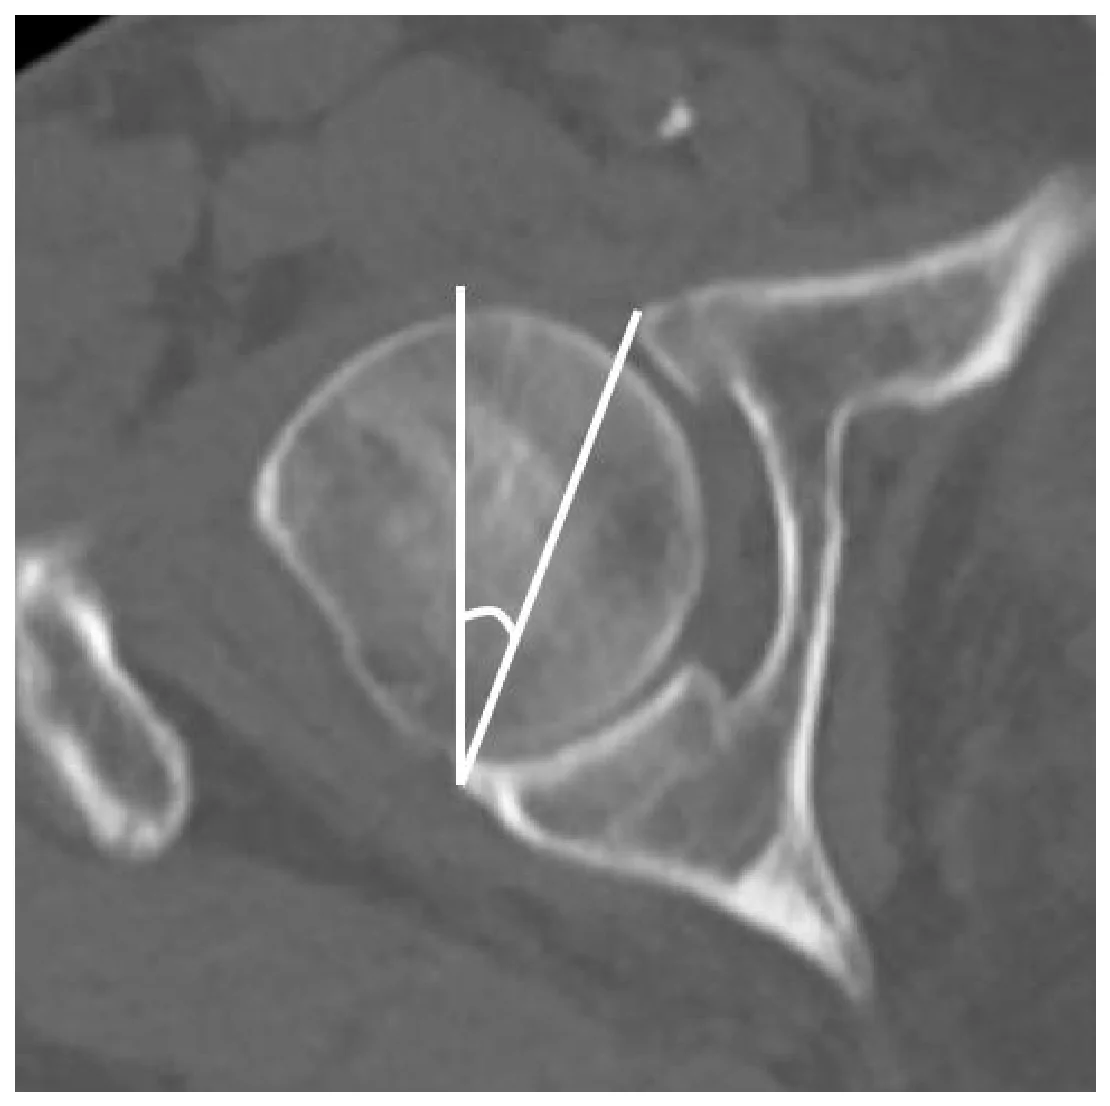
Acetabular anteversion measured on a mid-axial CT image.
